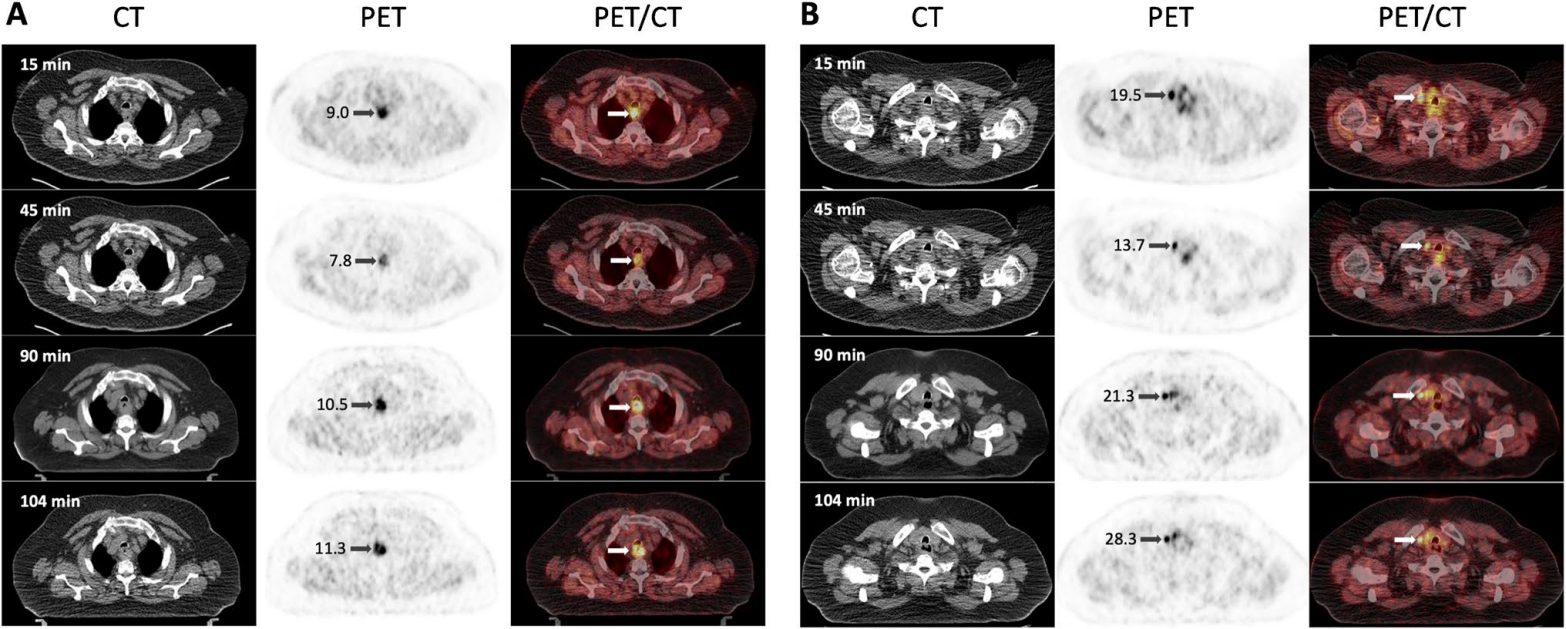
^68^Ga-D0103 PET/CT of a patient with esophageal squamous cell carcinoma (ESCC; f, 68 y, 115 kg, 193 MBq). PET is scaled to SUV 7 in all images. A, axial slices through primary tumor. B, axial slices through lymph node metastasis. Times indicate scan starts after tracer injection (p.i.). Numbers on arrows indicate the SUV_max_
